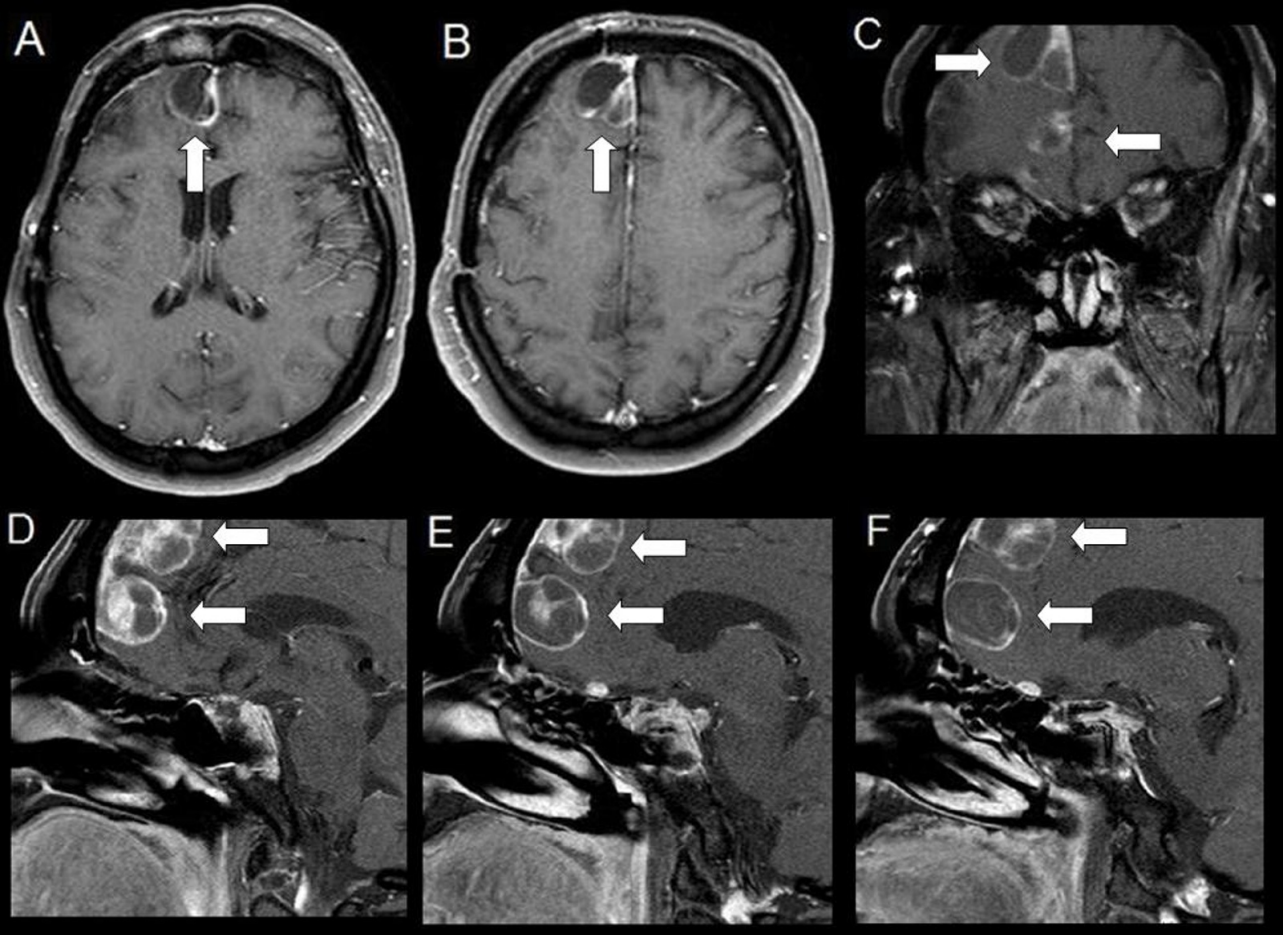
Contrast Enhanced Brain Magnetic Resonance Imaging (MRI) Two Years After Discovery of the Right Frontal Lesions, and Five Years After Initial Craniotomy Axial (A, B), Coronal (C), and Sagittal (D, E, and F) images showed peripherally enhancing, cystic structures along the anterior parafalcine and cribriform regions of the right front lobe. There was stable, slight residual enhancement of the sella turcica, and right cavernous sinus and carotid artery. The parenchyma was otherwise normal.
